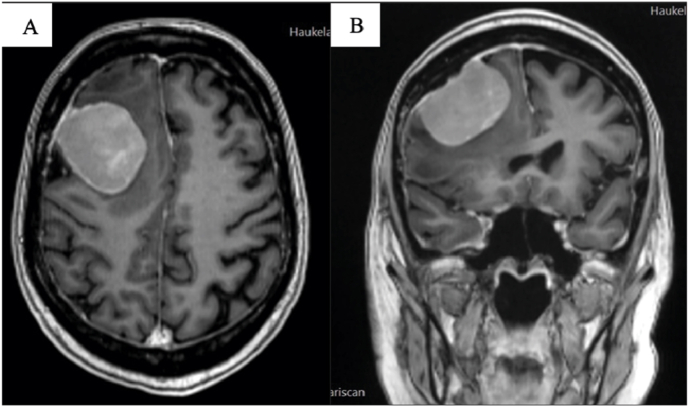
Contrast-enhanced T1-weighted images before surgery. A: MRI in axial plane. B: MRI in coronal plane.
